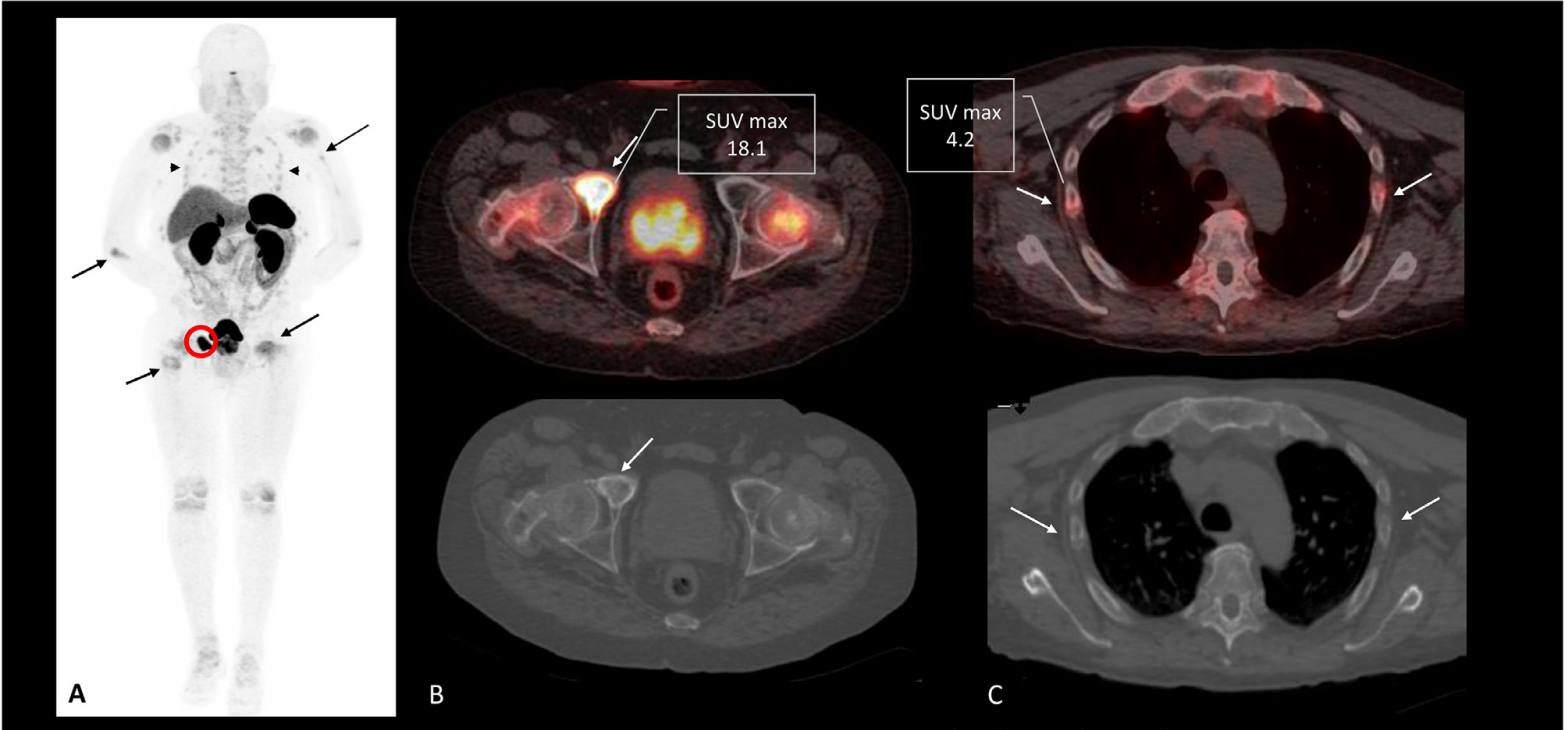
Ga-68 Dotatate PET-CT scan—(A) MIP image demonstrates multiple radiotracer avid looser zones (insufficiency fractures) involving the multiple ribs (arrowhead), left humerus, right ulna, and both proximal femurs (arrows). It also shows a somatostatin-receptor-rich lesion in the right anterior acetabulum (biopsy-proven phosphoturic mesenchymal tumors) with an SUV max of 18.1. (B) PET/CT axial fused and CTAC images show the sclerotic acetabular lesion (PMT) with an SUV max of 18.1. (C) PET/CT axial fused and CTAC images demonstrate the rib fractures (arrows) with SUV max (4.2) much lower than that exhibited by the primary acetabular mass.
